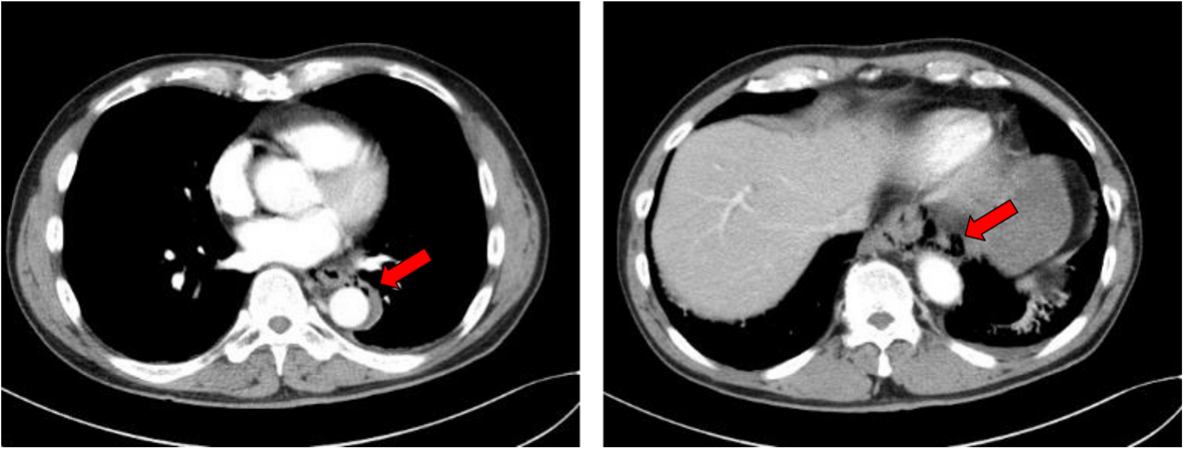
Computed tomography (CT) scan. CT revealed pneumomediastinum extending from the upper surface of the diaphragm to the aortic arch
